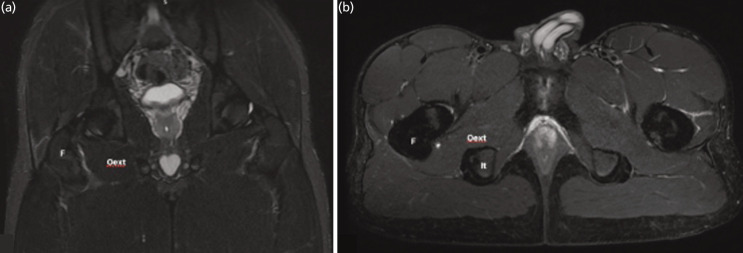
Control MRI performed after 35 days (STIR and PD fat sat images). In panel A (coronal, STIR) and B (axial, PD fat sat), MRI images demonstrate complete healing of OE. No area consistent with muscular tear can be observed. (F: femur; It: ischial tuberosity; Oext: obturator externus).
